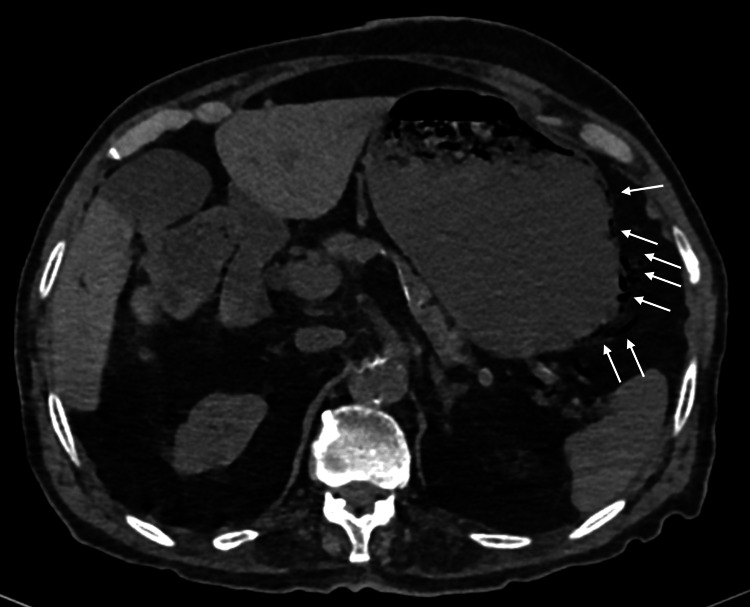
Non-contrast computed tomography (CT) demonstrating emphysematous gastritis with portal and mesenteric venous gas Non-contrast CT images show intramural gas within the posterior gastric wall, with hepatic portal venous gas and gas within mesenteric vessels. There is mild mural thickening at the splenic flexure/transverse colon with the dilatation of the colon and terminal ileum, consistent with ileus. No pneumoperitoneum, free intraperitoneal fluid, or drainable intra-abdominal collection is identified
